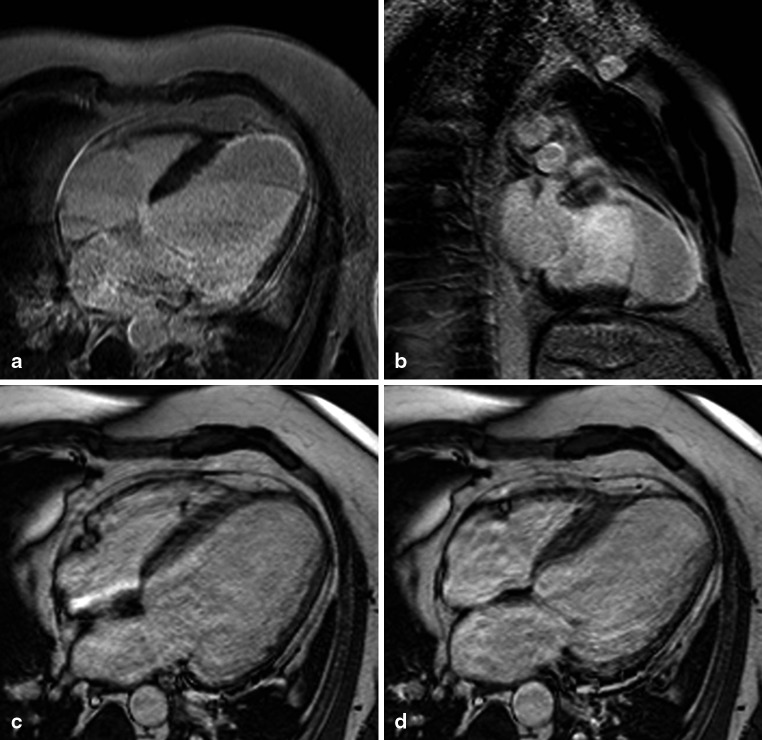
Late gadolinium enhancement (a, b) and cine (four-chamber view in end-diastole (c) and end-systole (d)) magnetic resonance images of a patient with a large myocardial infarction (left ventricular ejection fraction 15 %) of the left anterior descending coronary artery (right ventricular ejection fraction 56 %)
